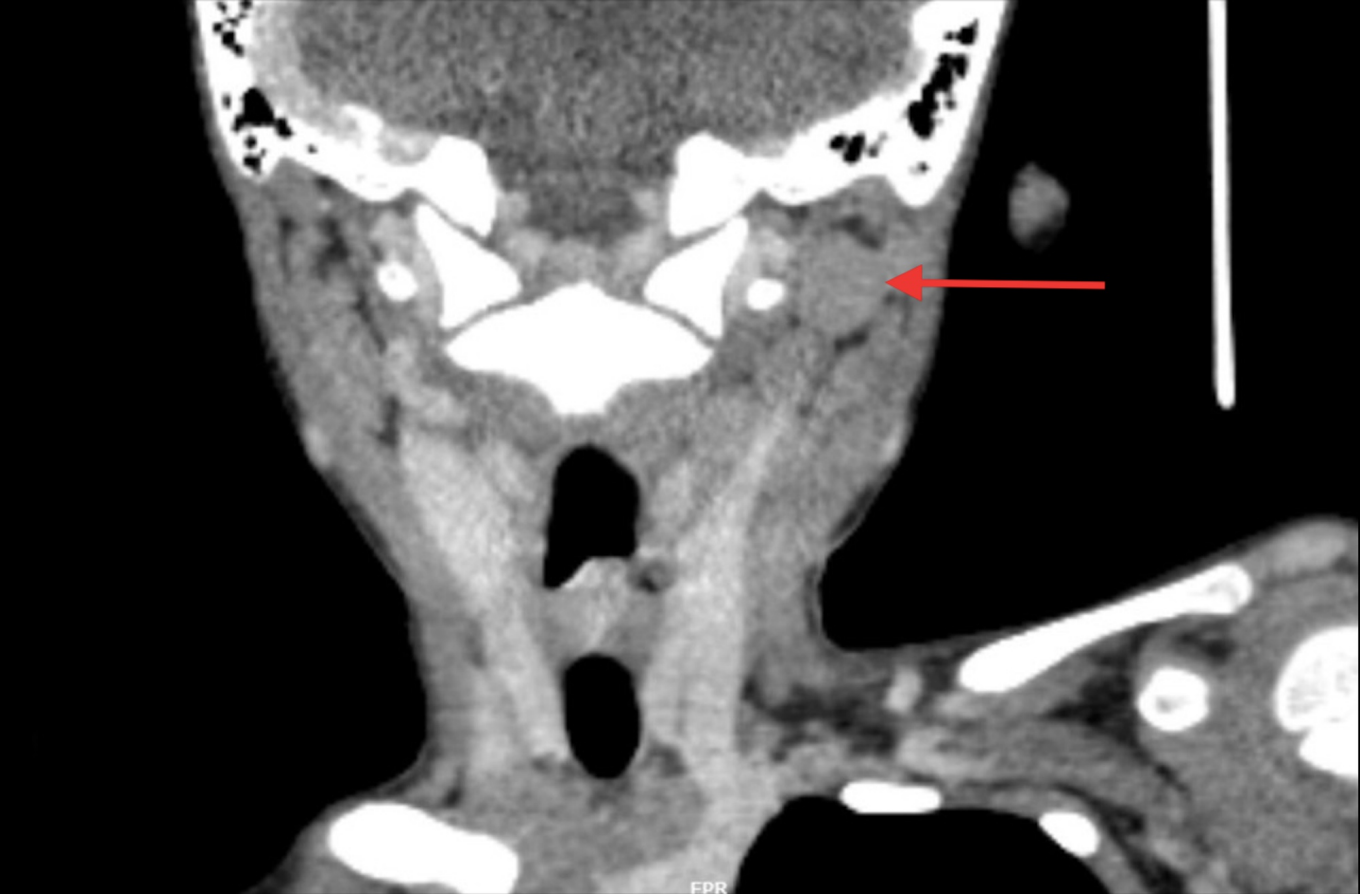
Coronal cut of CT showing multiple enlarged cervical nodes on the left side of the neck (Red arrow).
